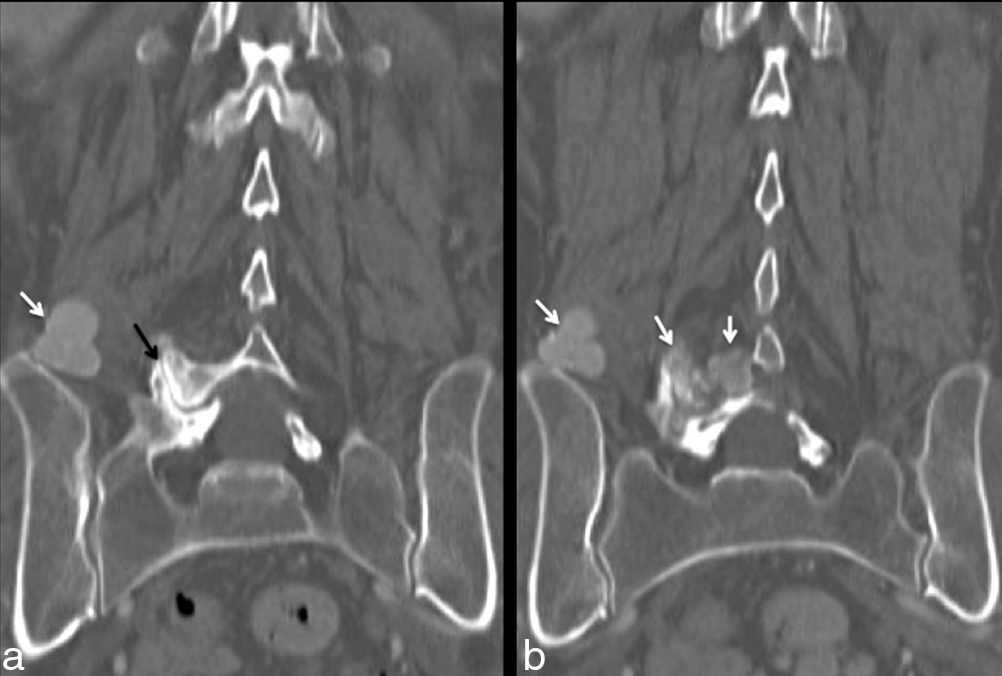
CT scan of the lumbar spine. Coronal reformatted CT images with bone window (a, b) showing calcified mass-like lesions in the right paraspinal soft tissues (white arrows). It also highlights the foraminal lesions centered on the right facet joint (b) that demonstrates severe degenerative joint disease (black arrow).
